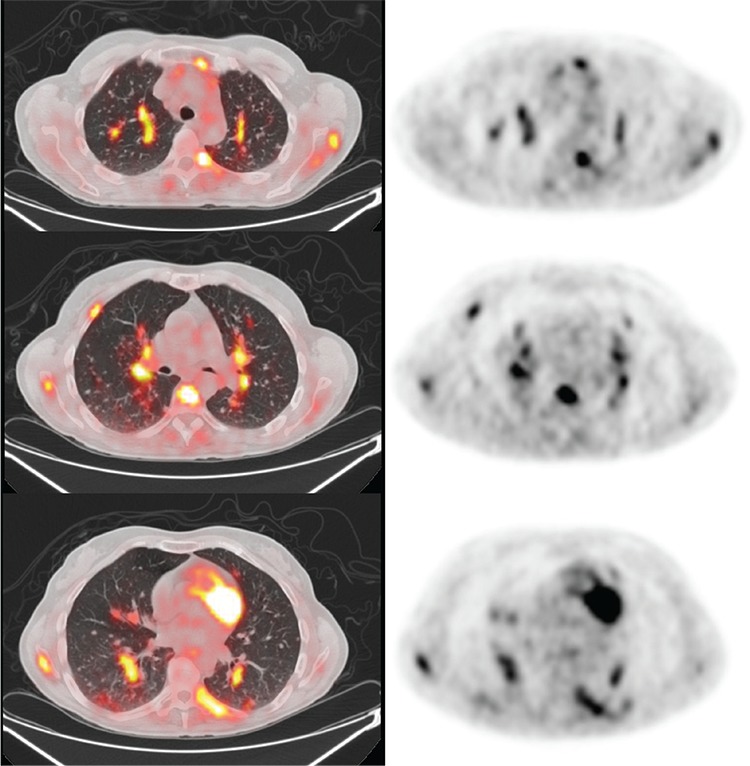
Intense ^18^F-FDG uptake was also noted in numerous peribronchovascular and subpleural nodules in both lungs (largest was 1.0 cm in diameter with SUV_max_ 3.2).
